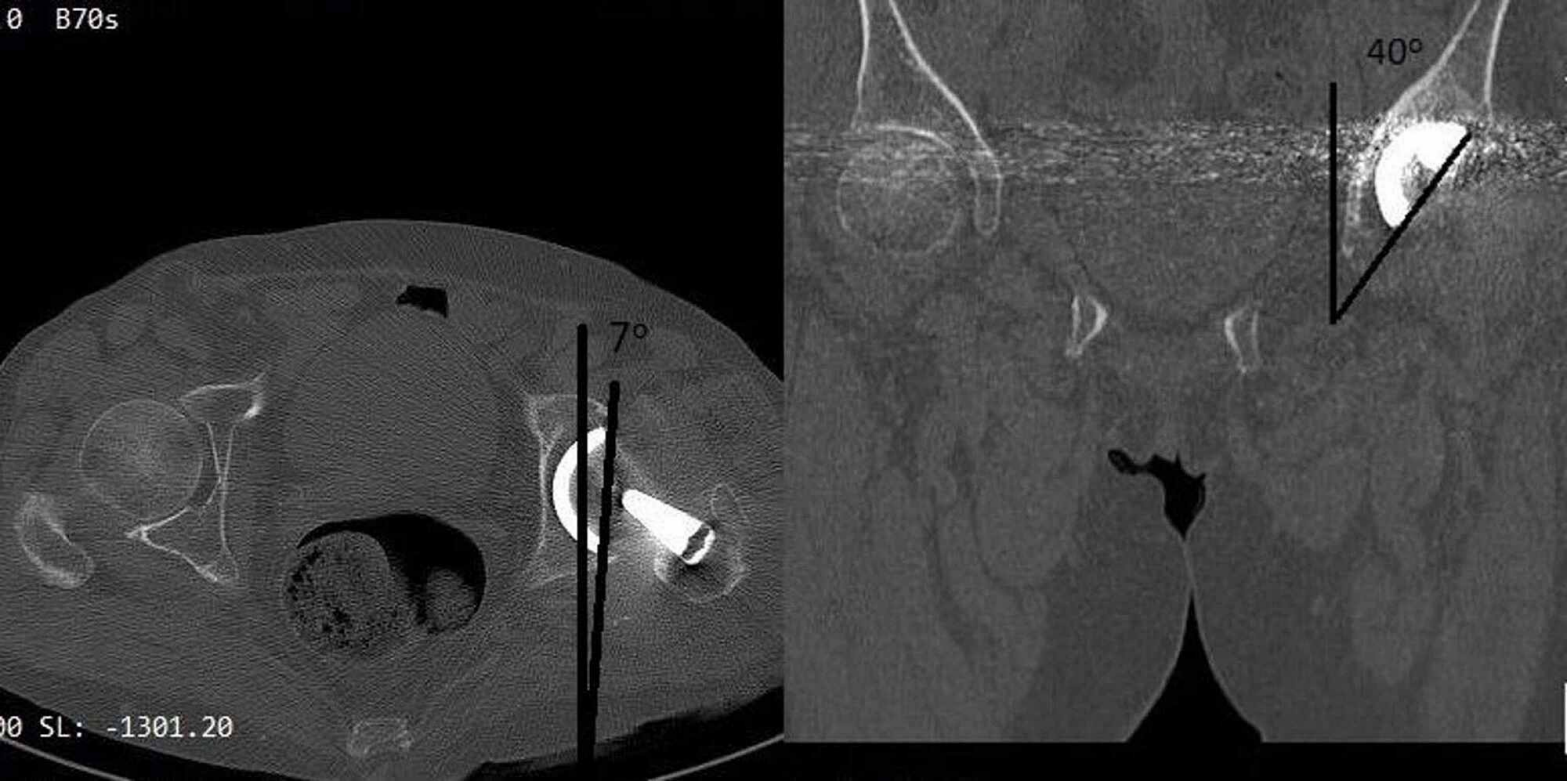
The left axial computed tomography image demonstrates the seven degrees of acetabular shell anteversion, while the right coronal computed tomography image indicates the 40 degrees of abduction
